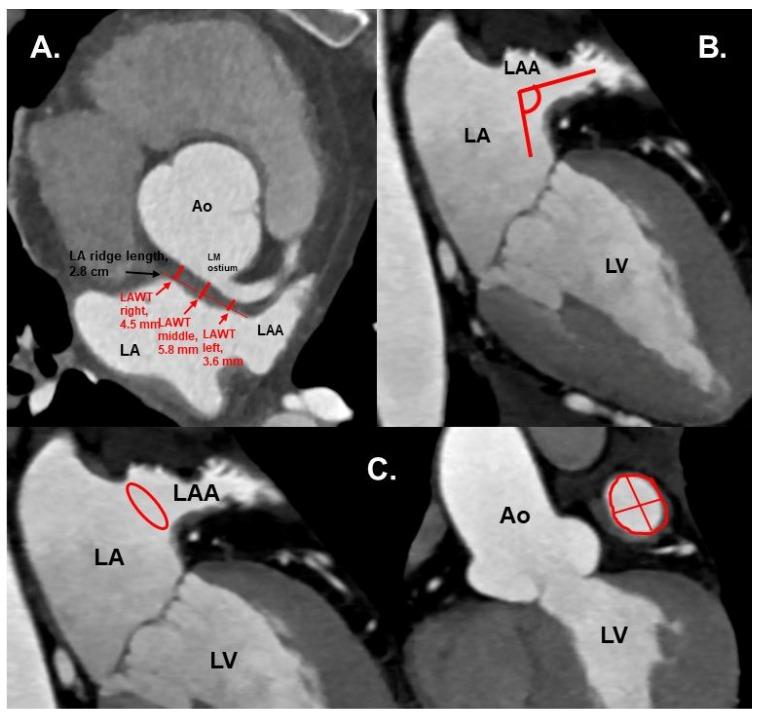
(A) Cardiac CTA: axial plane. Red arrows show the LAWT measurements at the left, middle and right points, and the LA ridge length. Measurements were taken at the plane of left main coronary ostium. (B) CTA: axial oblique plane, showing LAA ostium angulation (red lines) to orthogonal plane. (C) CTA with axial oblique planes of the heart. Left: LAA ostium area shown in red circle. Right: LAA ostium area (red circle), length and width (red lines). Ao—aorta, LA—left atrium, LAA—left atrial appendage, LAWT—left atrial wall thickness, LM—left main coronary artery, LV—left ventricle.
